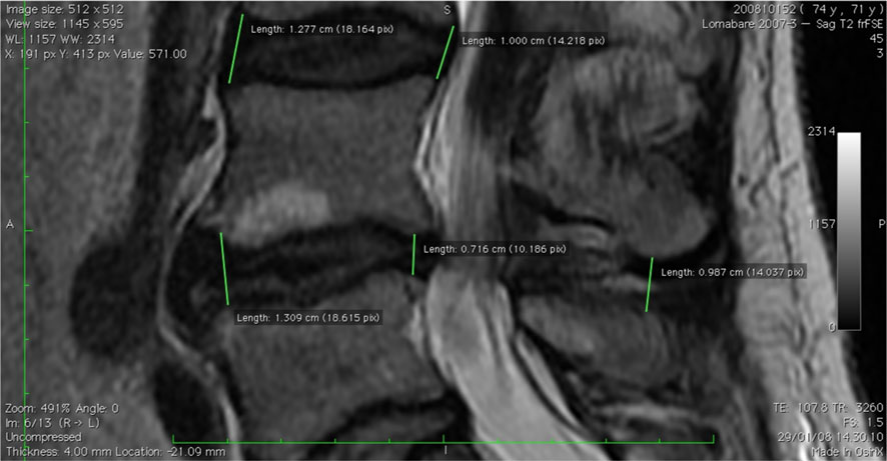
MRI preoperative measurement
